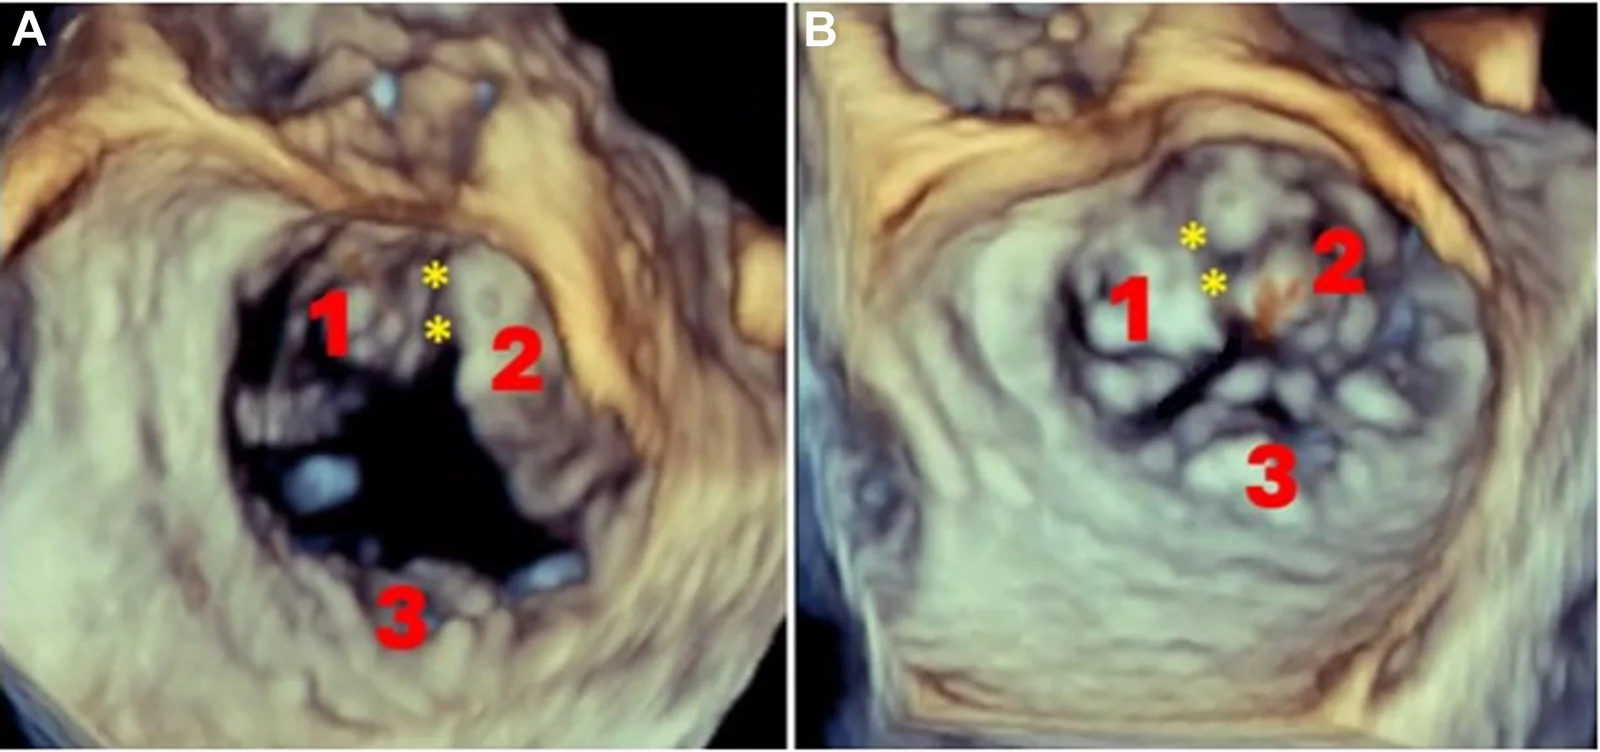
Three-Dimensional Transesophageal Echocardiographic En Face Views of the Mitral Valve Demonstrating a Trileaflet-Appearing Morphology (A) Mitral leaflet anatomy in diastole. (B) Leaflet anatomy in systole. Labels 1, 2, and 3 denote the trileaflet morphology with 3 distinct leaflets in the setting of a congenital mitral cleft, denoted by yellow asterisks.
